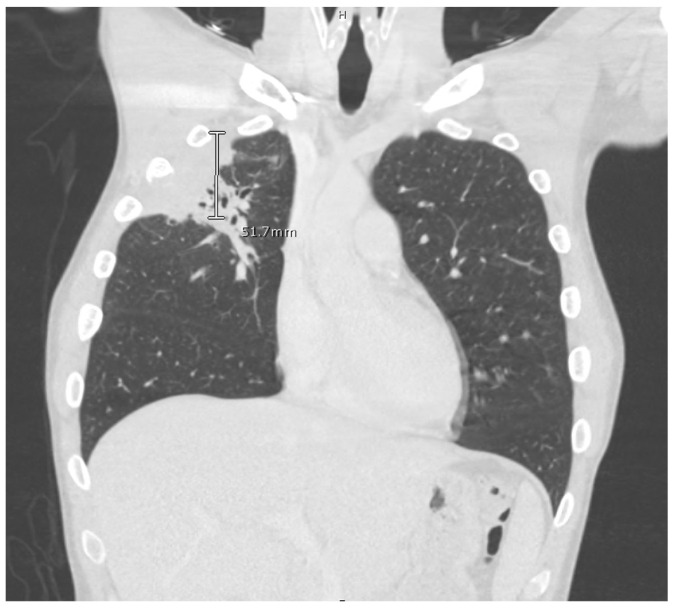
CT scan of chest, right upper lobe pleural and subpleural consolidation with erosive changes at the 3rd and 4th ribs and involvement with the chest wall.
